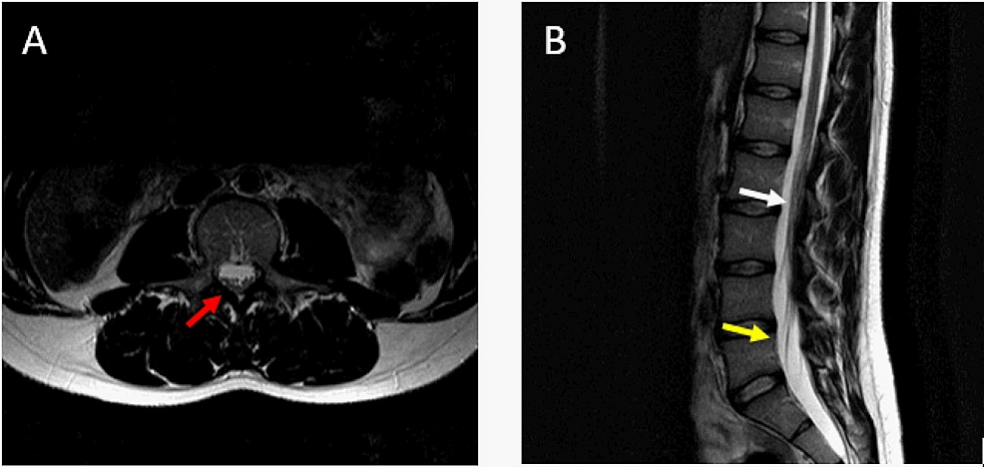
Patient’s lumbar MRI The first picture (A) corresponds to a transverse MRI view. We can observe fibrotic strands in the posterior aspect of the spinal cord (red arrow). Image B is a sagittal cut of the same MRI, the white arrow points to the section of the spine that is tethered, and the yellow arrow points toward the unusual low-lying cone.
